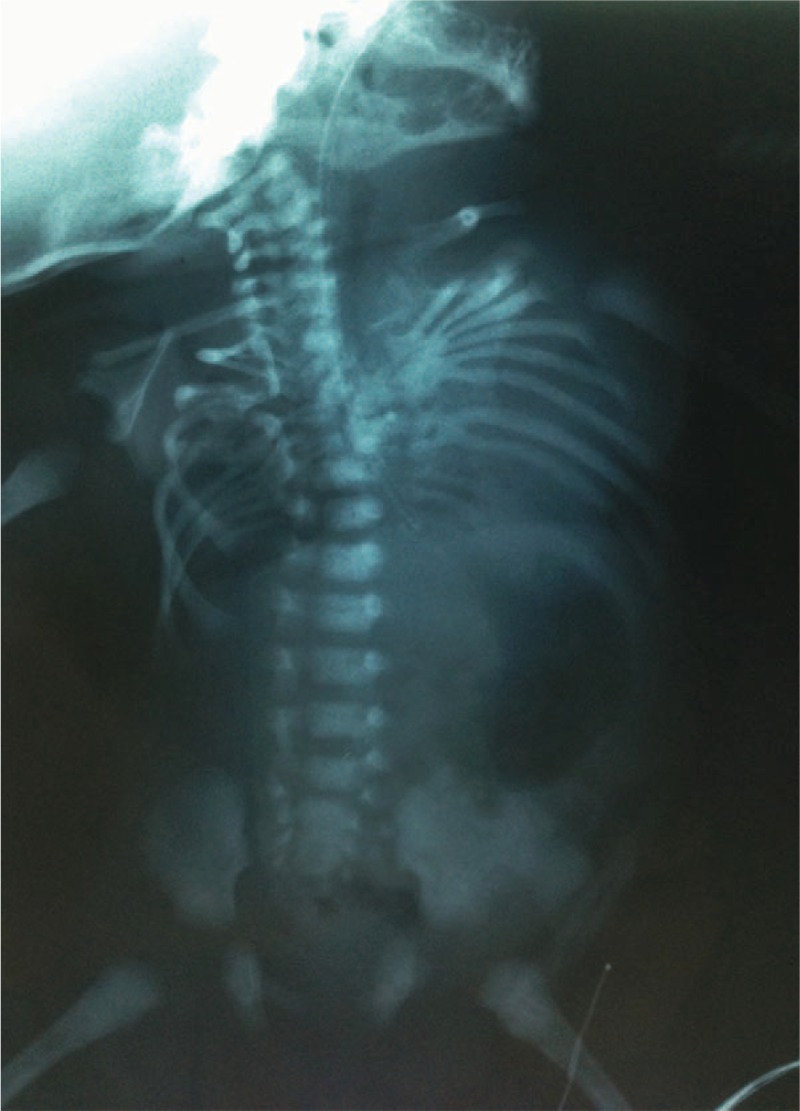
Radiograph of case 11 shows multiple abnormalities of the thoracic vertebrae with hemivertebrae, fused vertebrae, and fusion and bifurcation of the ribs. Segmentation anomalies appear throughout the majority of thoracic spine. Thorax is asymmetrical, and has mild scoliosis. The right lung is somewhat smaller than the left. Note that despite multiple vertebral segmentation defects, a “crab-like” thoracic spine is not evident. The radiograph shows asymmetrical abnormalities of the vertebral bodies and ribs, but not the symmetrical posterior fusion of the ribs so characteristic of spondylothoracic dysplasia.
